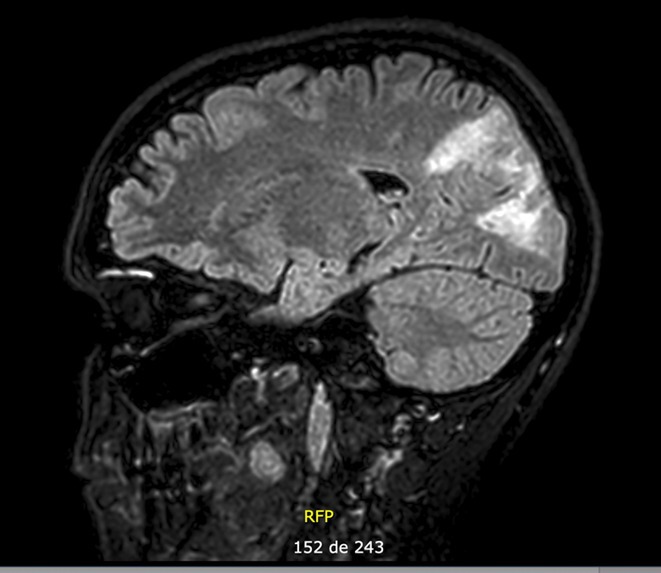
FIGURE 1 Brain MRI in sagittal flair
